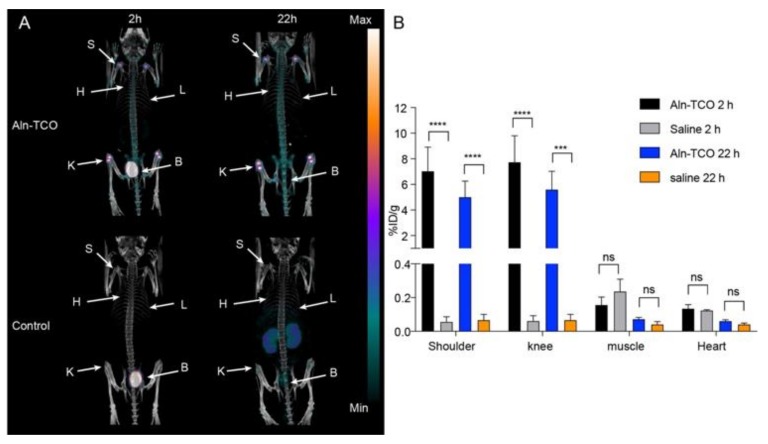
Pretargeted SPECT/CT bone imaging of [^111^In]2 targeting TCO functionalized alendronate (4), which was administered 1 h prior to the administration of [^111^In]2. (A) Respective SPECT/CT images after 2 h and 22 h. (B) Image-derived quantitative analysis of tissue uptake. *, *p* < 0.001; and , *p* < 0.0001. Each image is scaled between its minimum and maximum pixel intensity.
